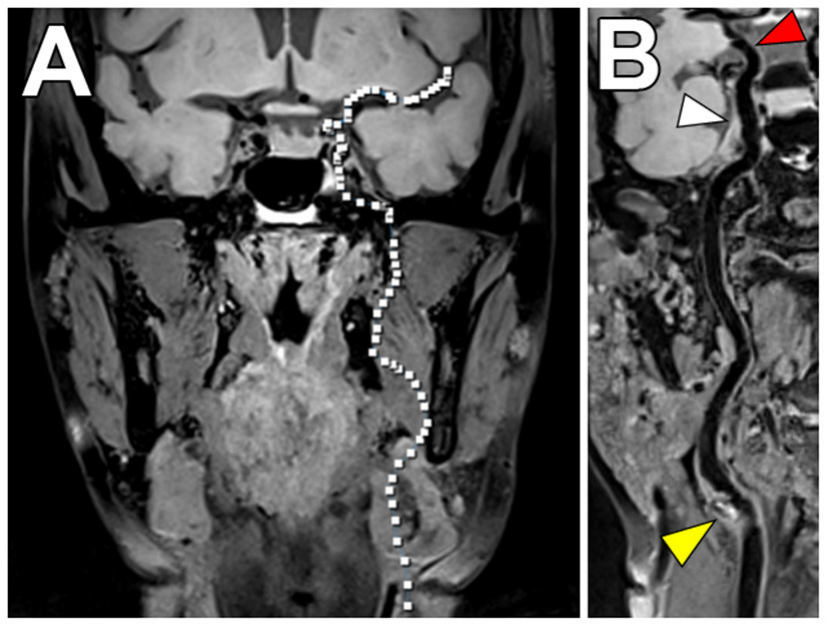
Joint VW-MRI of the extracranial and intracranial arteries. (A) Coronal precontrast VW-MRI image shows the coverage of the joint intracranial and extracranial VW-MR image. (B) A curved reformatted image of the left cervico-cranial carotid artery shows intrinsic T1 hyperintense signal at the carotid bulb (yellow arrowhead), which was favored to be the culprit source of plaque in this patient with ischemic strokes. The intracranial internal carotid artery at the siphon (white arrowhead) and M1 middle cerebral artery segment (red arrowhead) showed no significant wall thickening to suggest intracranial atherosclerosis.
